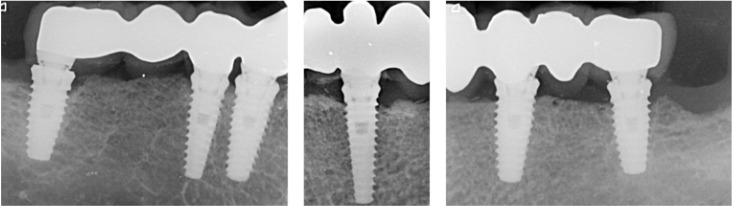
Radiographic assessment at the 3-month follow-up.
